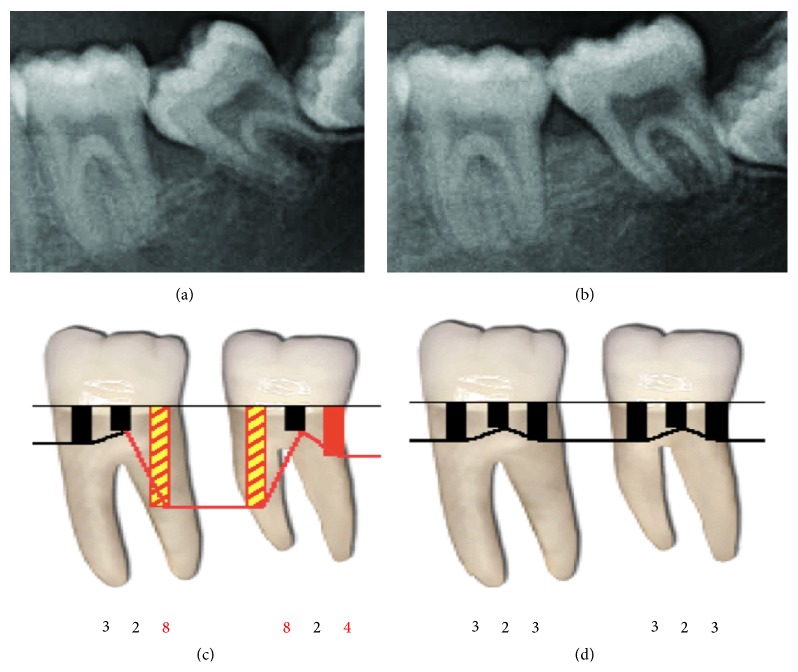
(a) Radiographic view of pretreatment, (b) radiographic view of third month after the treatment, (c) probing pocket depth values of pretreatment, (d) probing pocket depth values after the treatment.
